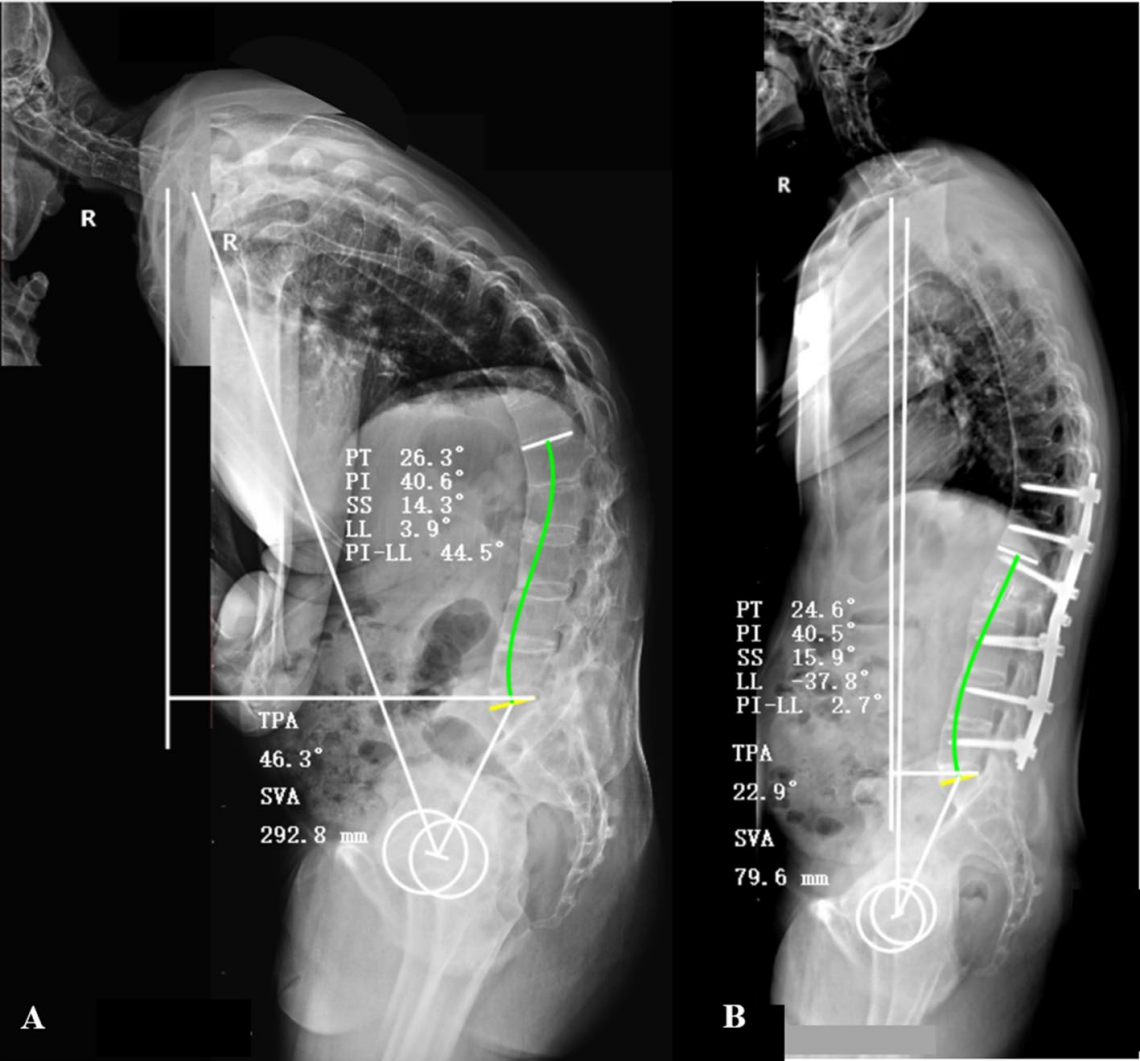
A 42-year-old man suffered from thoracolumbar kyphosis secondary to ankylosing spondylitis. (A) Preoperative radiographs showed severe global kyphosis and sagittal imbalance. (B) The patient underwent one-level three-column osteotomy on L2, following which the kyphosis was corrected and the sagittal alignment was restored.
